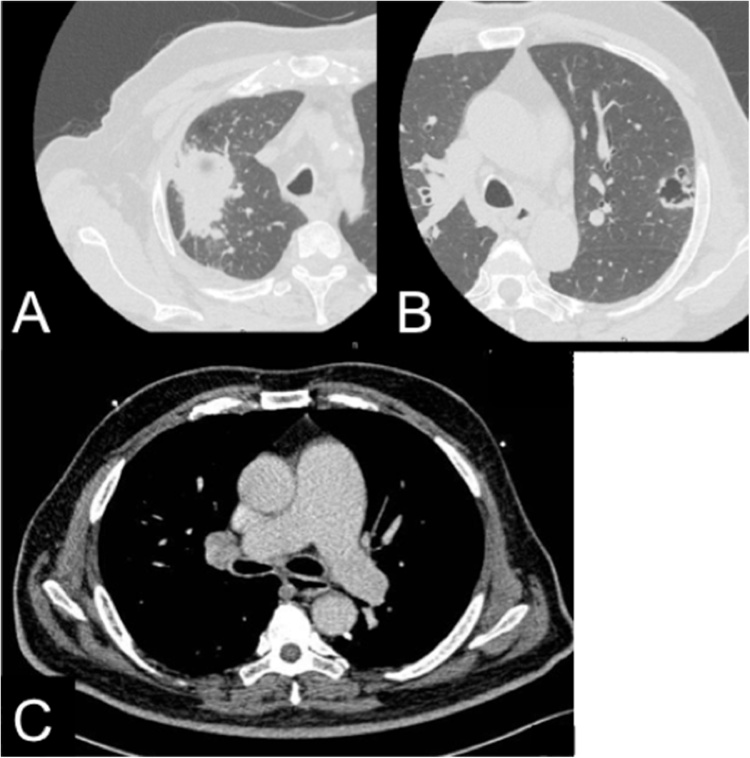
(A) Thoracic computed tomographic scan showing a 5.2 × 4-cm mass lesion in the right lung. (B) A 3.1 × 2.5-cm cavitating lesion in the left upper lobe. (C) Contrast-enhanced computed tomography image showing an enlarged hilar lymph node.
